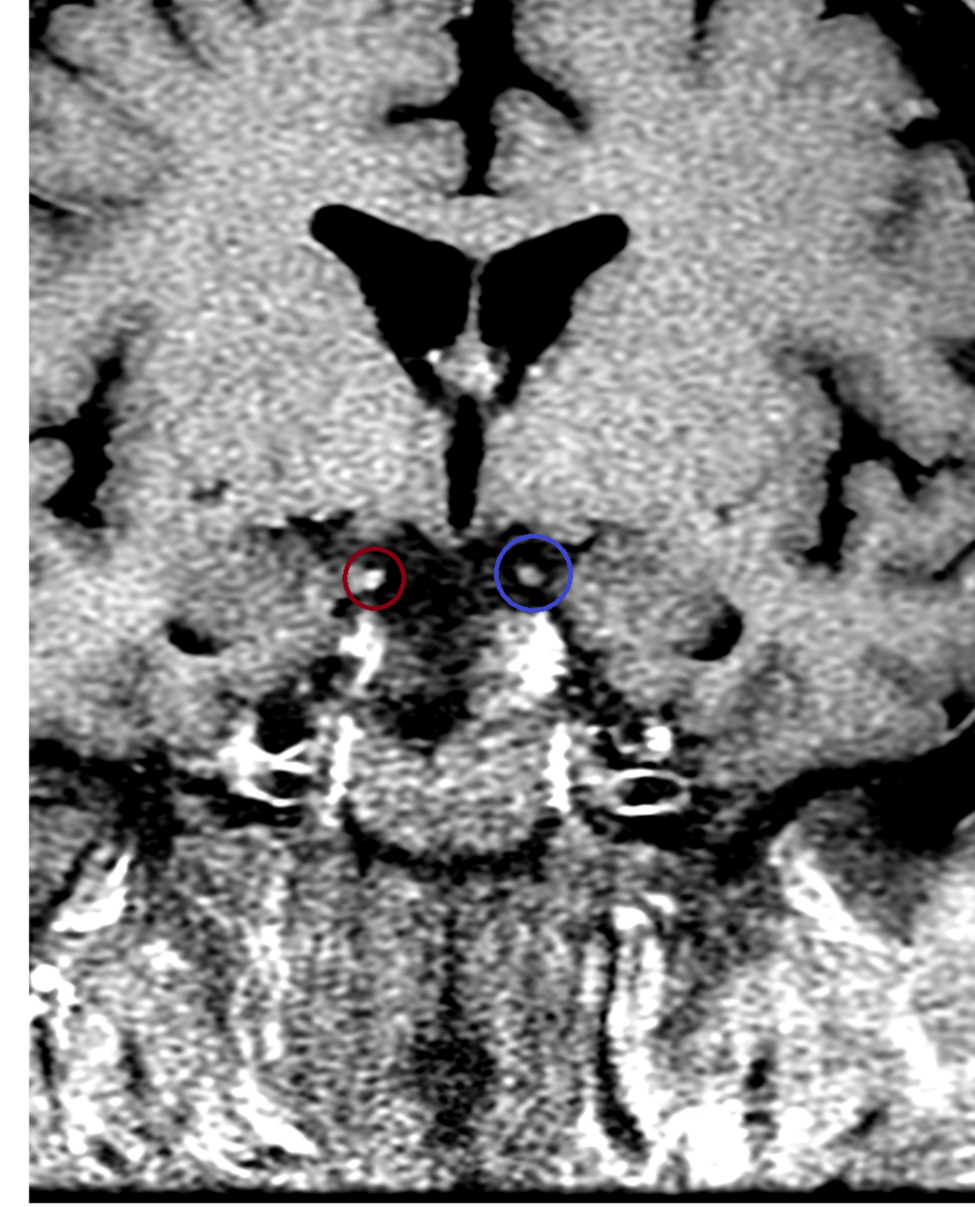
MRI Head T1, Coronal Section. In the figure the red circle shows abnormal enhancement of right CN 3 and blue circle shows normal left CN 3 CN: cranial nerve
